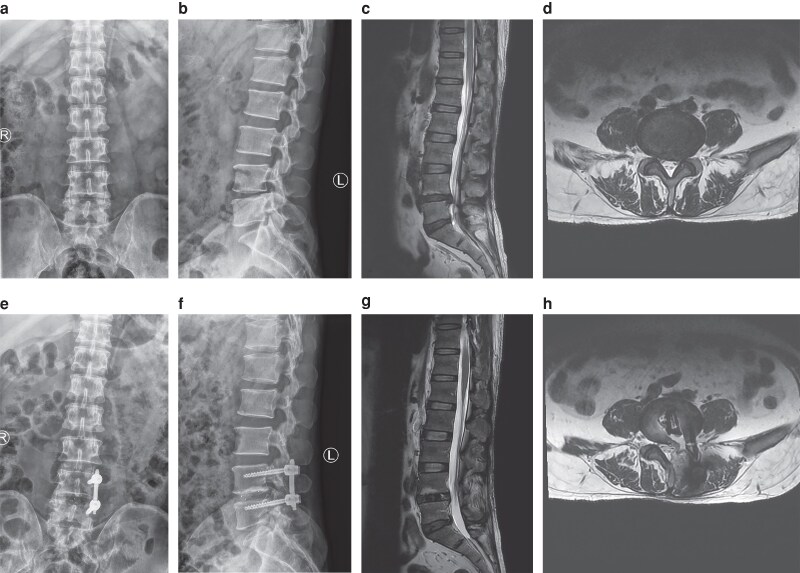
A 56-year-old male with lumbar disc herniation who underwent L4-5 unilateral biportal endoscopic-transforaminal lumbar interbody fusion a, b preoperative anterolateral X-ray; c preoperative T2 sagittal MRI showed L4-5 herniation; d preoperative T2 axial MRI; e, f postoperative anterolateral X-ray; g preoperative T2 sagittal MRI; h preoperative T2 axial MRI. MRI, magnetic resonance imaging.
